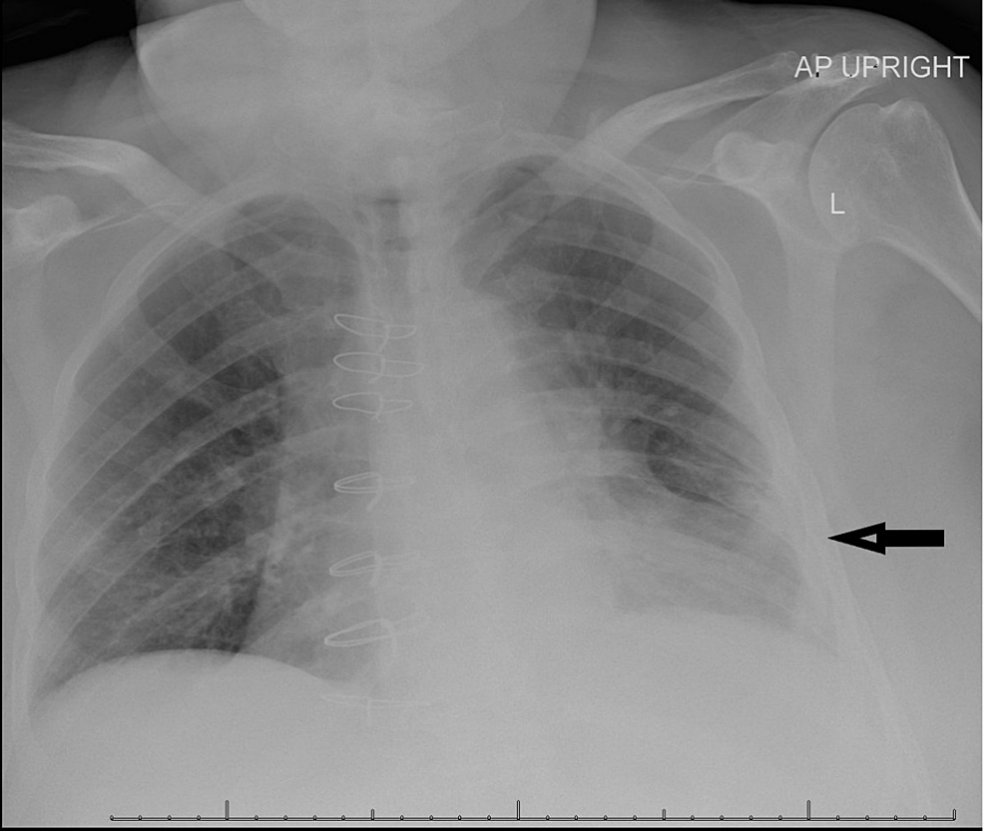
Chest radiograph showing left peripheral lower zone pleural thickening versus non-layering pleural effusion.
